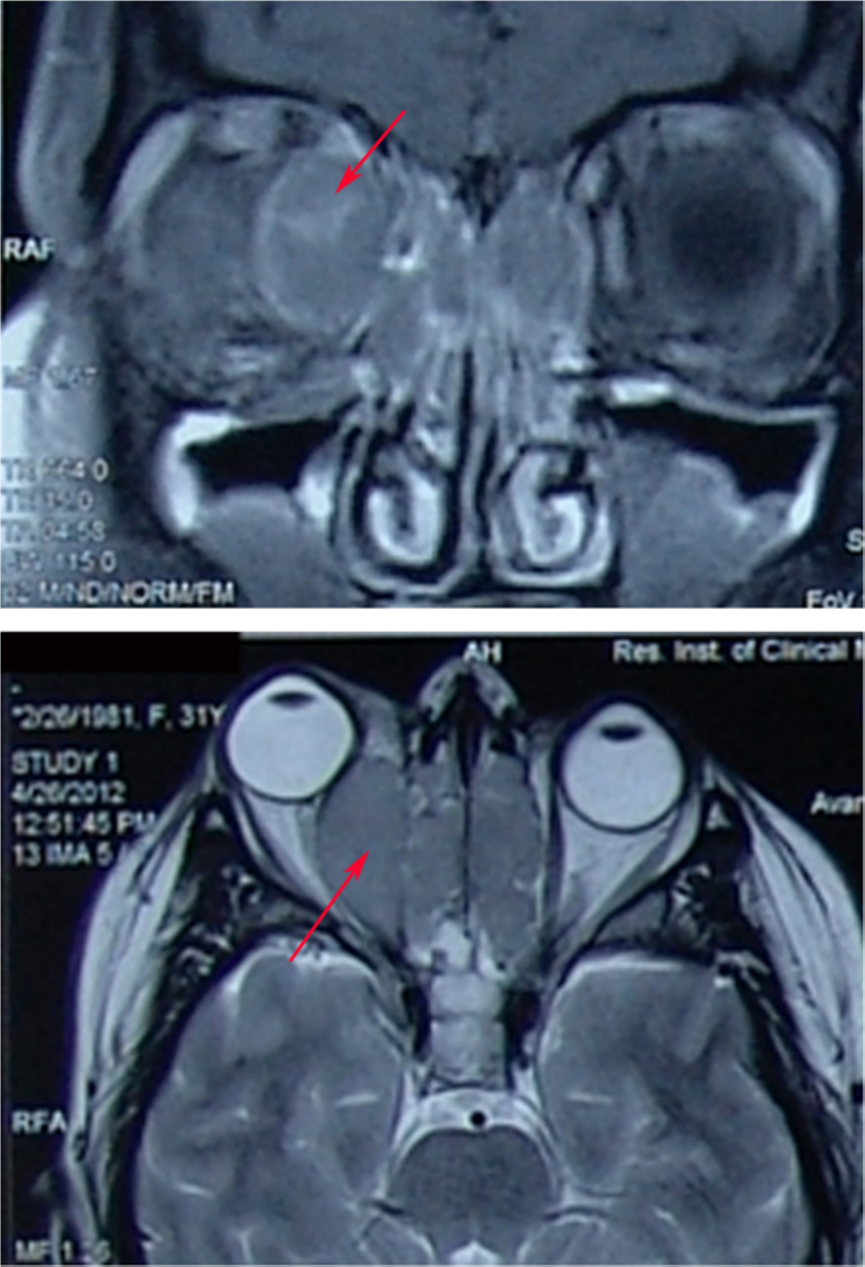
Brain magnetic resonance imaging and angiography of the cranial vessels using intravenous contrast agent shows right-sided proptosis and an oval structure with highly dispersed protein content (red arrow).
